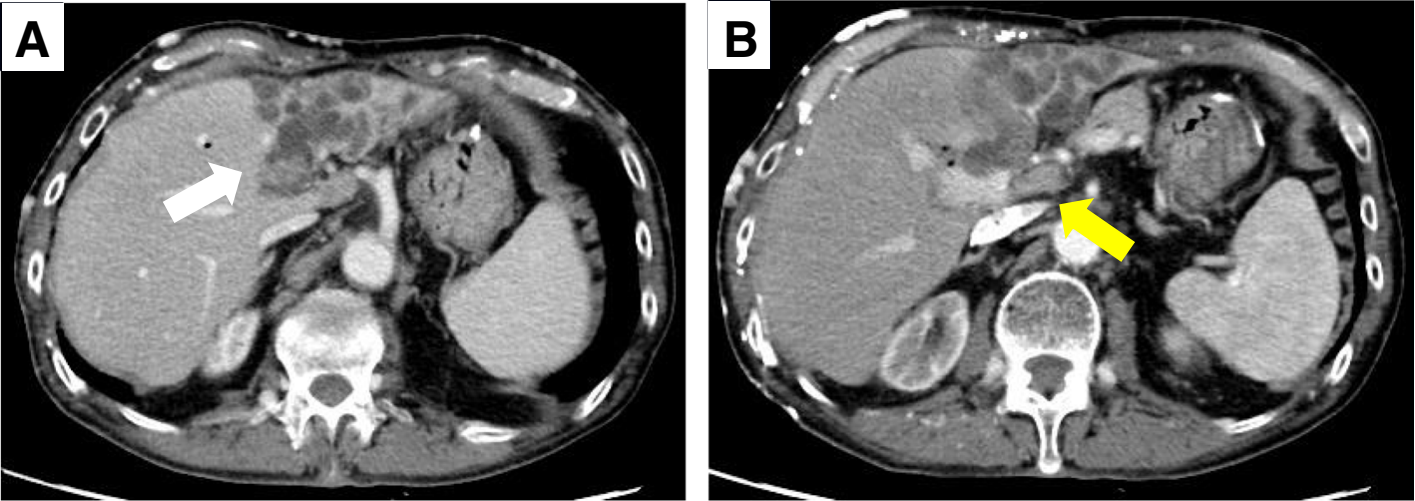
Preoperative enhanced computed tomography. Abdominal axial enhanced computed tomography showed a mass with a rich contrast effect in the left hepatic duct and diffuse dilation of the left intrahepatic bile duct (white arrow) (a) and a 12-mm lymph node in the hepatic portal region (yellow arrow) (b)
